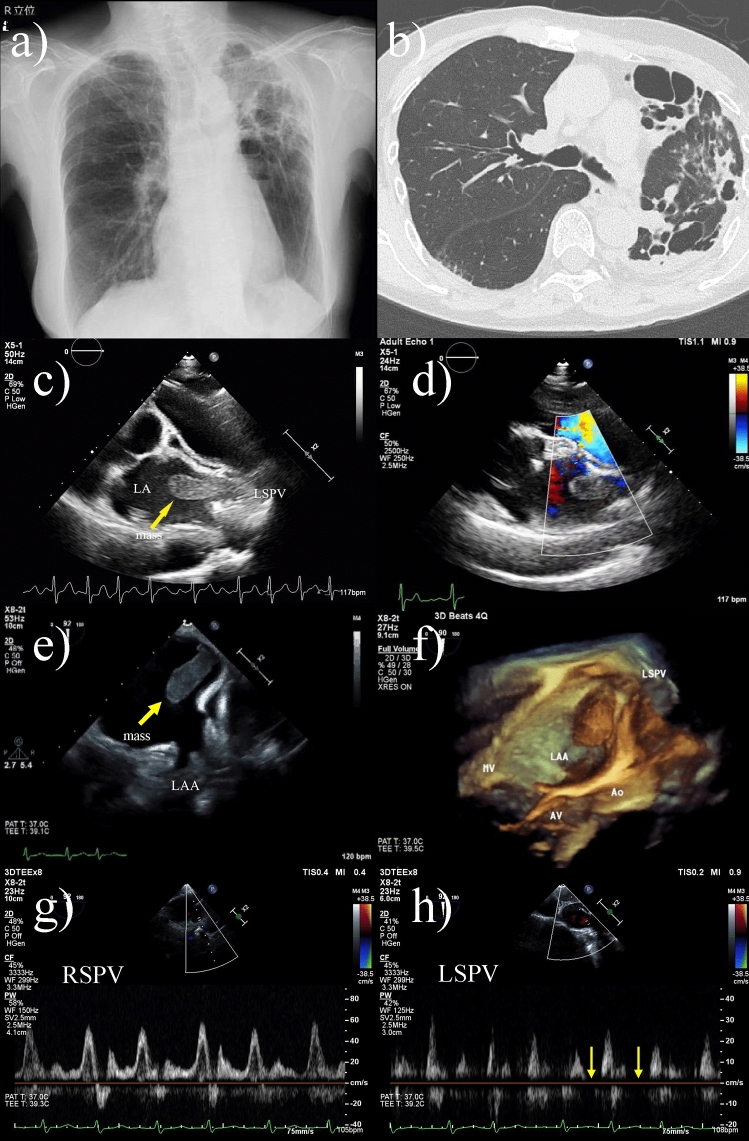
a Chest radiograph showed a cardiothoracic ratio of 49% and no cardiac enlargement, with abnormal shadows mainly in the left superior lung field and decreased air content in the left lung. b Chest computed tomography showed destruction of the structures of the left superior lobe of the lung. c Transthoracic echocardiography shows a mass protruding from the left superior pulmonary vein (LSPV) to the left atrium (LA) (arrows). The mass measures 32 × 12 mm and has a well-defined margin and an isoechoic and heterogeneous inside structure. d Color Doppler imaging confirms that there is no blood flow signal inside the mass. e Transesophageal echocardiogram (TEE) shows a mass (29 × 11 mm) attached to the LA wall at the LSPV orifice (arrows). f 3D-TEE showed that the thrombus adhered to a site distinctly different from the left atrial appendage, and was observed to be club-shaped from the LSPV to the LA. g, h Normal pulmonary vein blood flow is continuous, but the LSPV blood flow in this case is intermittent, with periods of cessation of blood flow (arrows). *MV* mitral valve, *AV* aortic valve, *Ao* aorta, *RSPV* right superior pulmonary vein
